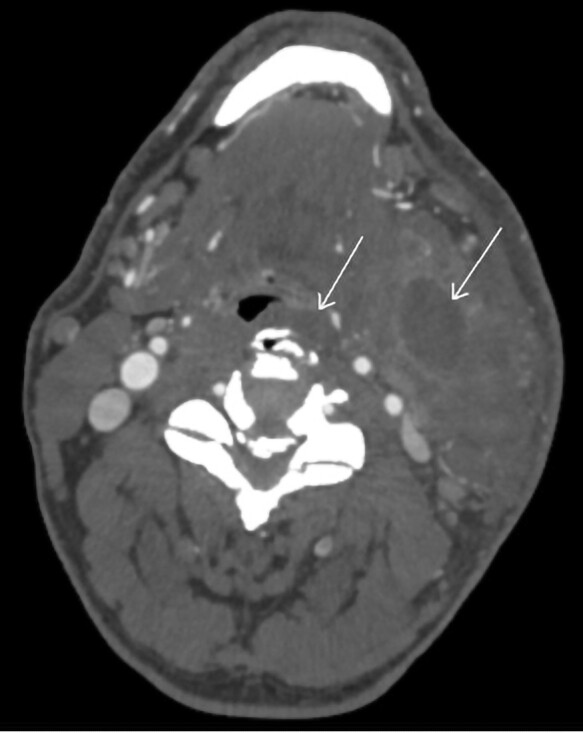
Computed tomography image of neck, demonstrating cervical retropharyngeal phlegmon (arrow on left) and abscess in the sternocleidomastoid muscle (arrow on right).
